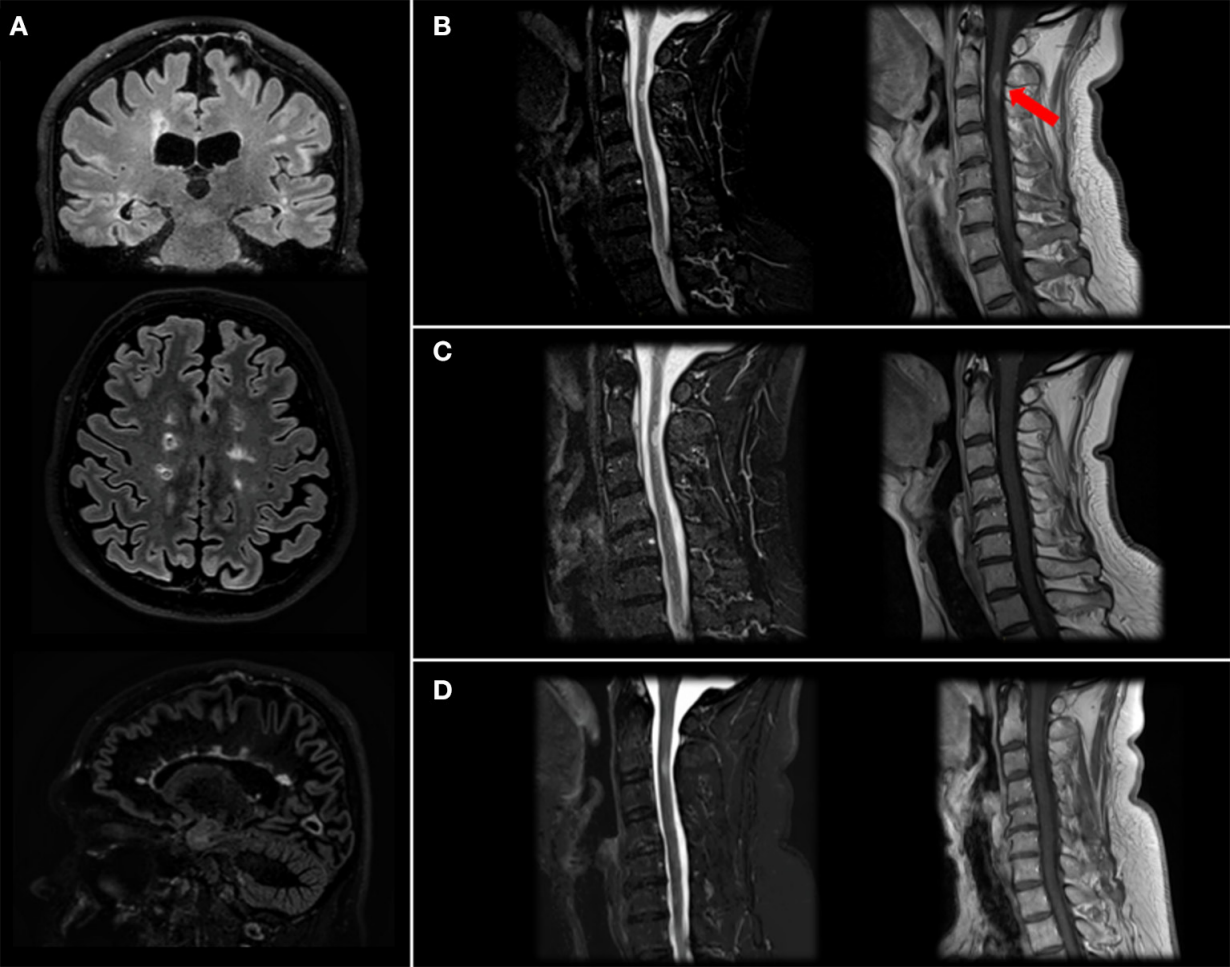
Brain and spinal cord MRI. Brain MRI images (Fluid Attenutated Inversion Recovery coronal and axial projections, Double Inversion Recovery sagittal projection) showing typical MS lesions involving bi-hemispheric white matter (A). Cervical MRI image (Short Time Inversion Inversion Recovery and T1-gd+ sagittal projection) at diagnosis (B) showing a C2-C3 level lesion with gd-enhancement (arrow), resolution after steroid treatment (C) and stability at 4-month follow-up after two cycles of Rituximab (D).
